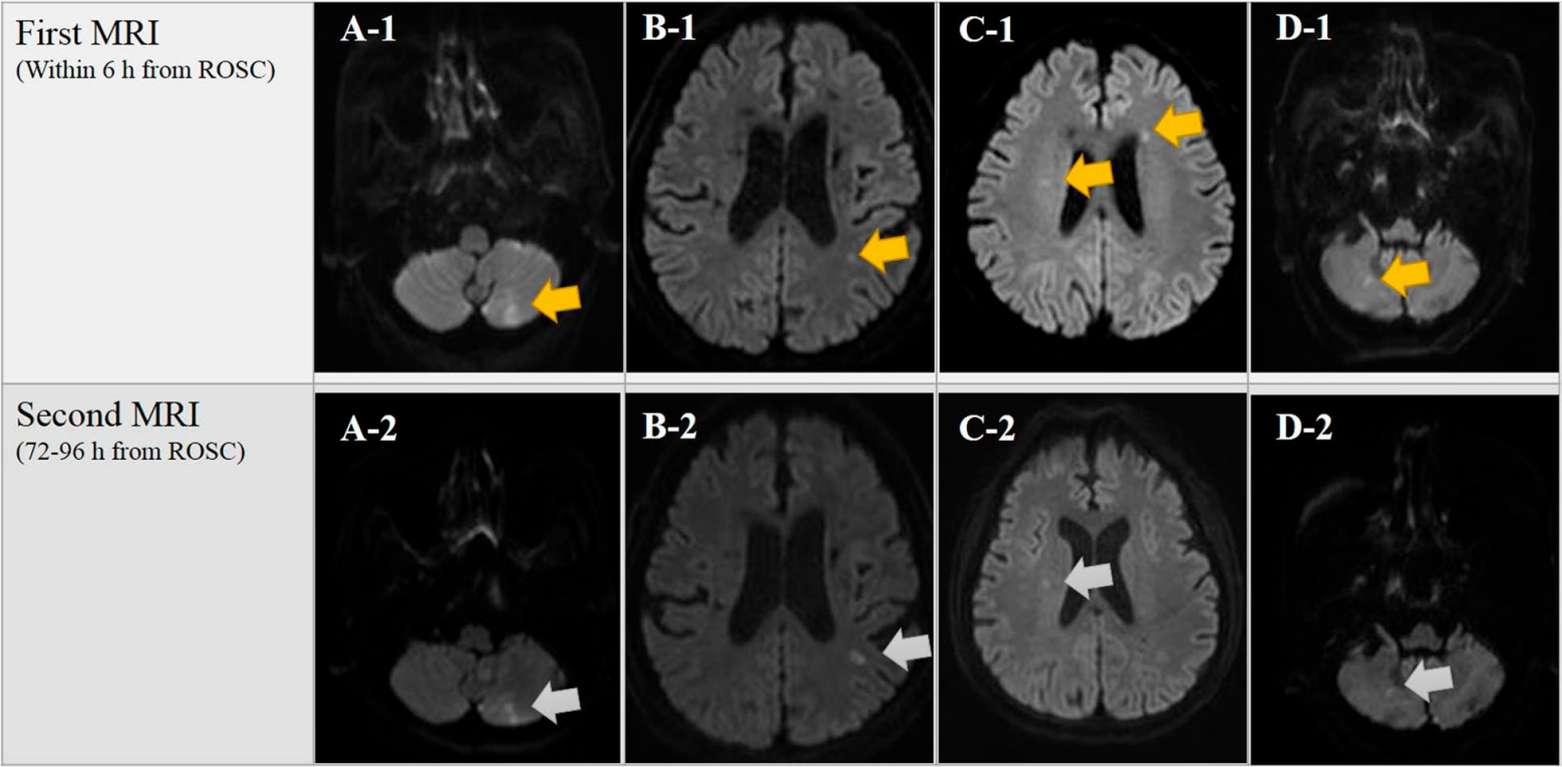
Patients excluded from this study despite DW-MRI showing the presence of HSI. Our institution's targeted temperature management protocol recommends but does not require obtaining two brain MRI scans within 6 h (first MRI) and between 72 and 96 h (second MRI) after ROSC. In this study, 4 patients showed only 1 or 2 focal HSI (orange arrow) on the ultra-early DW-MRI, and all of them did not exhibit an expanded HSI (gray arrow) area in the DW-MRI 3–4 days after ROSC. They all showed good neurological outcome, and among them, cases A, B, and D showed higher CPC scores (CPC 1) compare with case C (CPC 2). *Abbreviations:*
